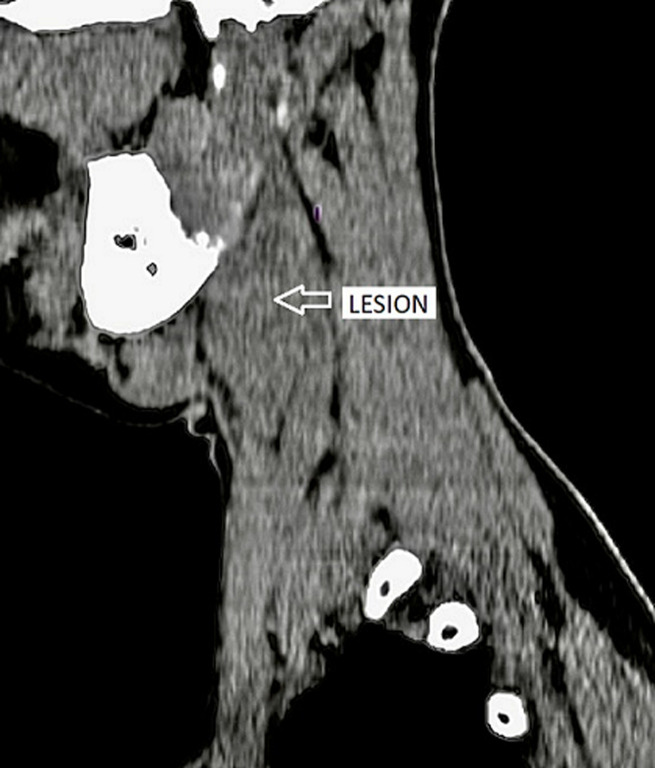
CT-scan of a bilobed soft tissue density mass lesion on the right side of the neck anterior to the sternocleidomastoid muscle (arrow)
